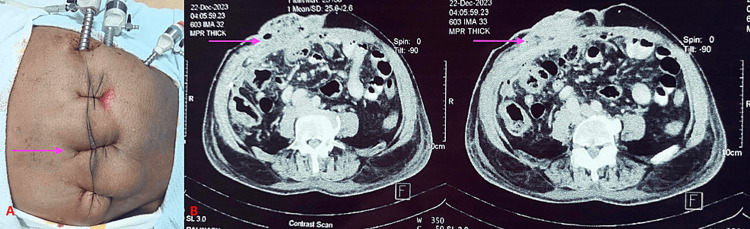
(A) The patient with a right subcostal incisional hernia defect closed with a transabdominal suture (Nylon no. 1 suture) (pink arrow), (B) CT scan showing the abdominal defect in the right subcostal region post-open cholecystectomy (pink arrows).
